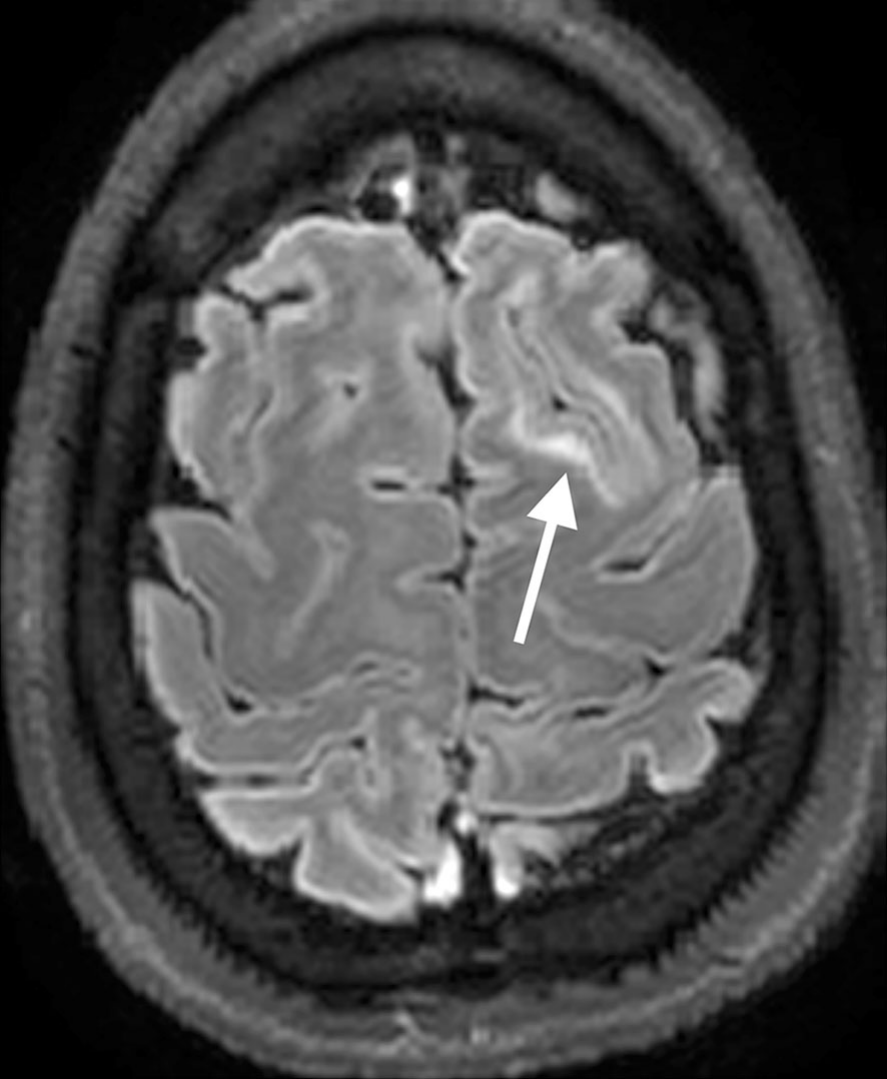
7 T FLAIR image, showing highlighted FCD
